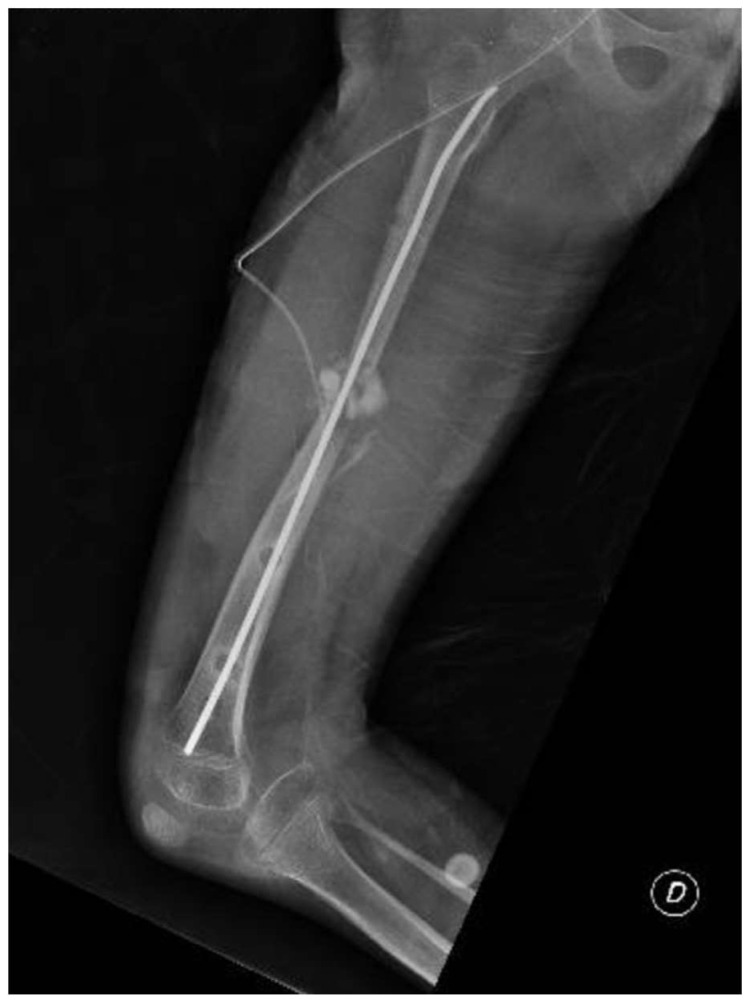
Radiographs showing Elastic Stable Intramedullary Nailing (ESIN) of the femoral fracture.
